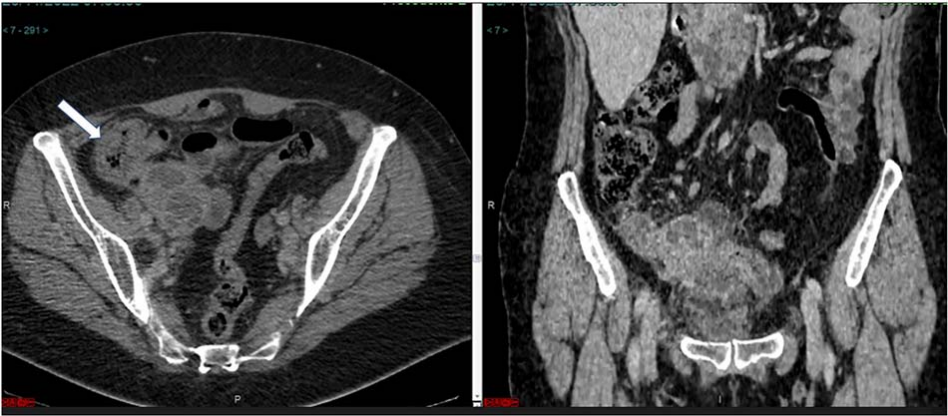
(a) Axial CT section of the abdomen showing signs of mucocele of the appendix with intraluminal air. (b) The coronal CT section of the appendix shows that the anterior wall of the appendix had an irregular thickening close to the transition of the middle/distal third.
